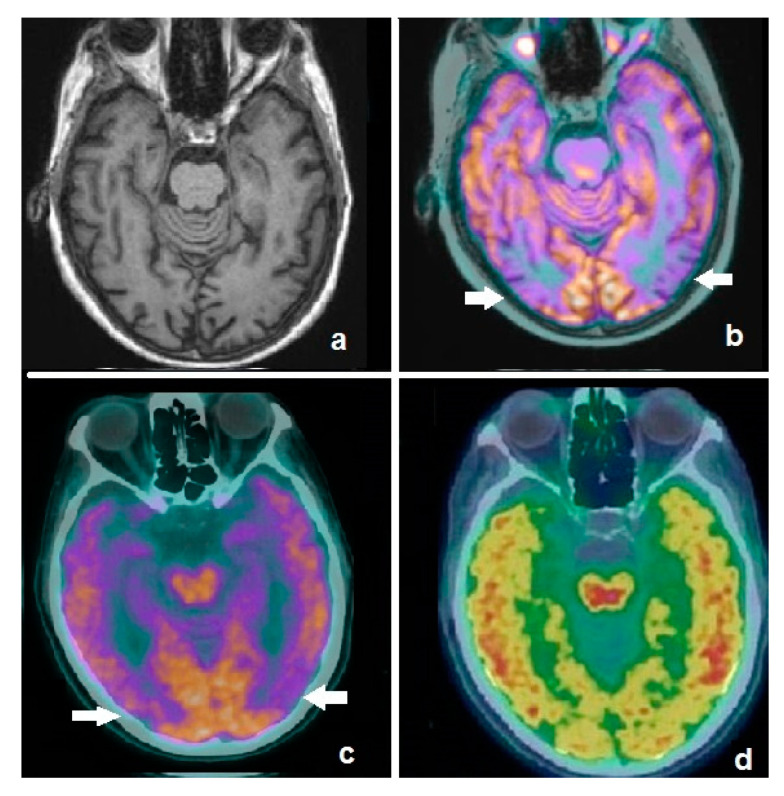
Axial image of an MRI scan in a patient affected by mild cognitive impairment (a), showing no significant abnormalities. Fused corresponding ^18^F-FDG PET/MRI demonstrates hypometabolism in temporo-occipital lobes (b, white arrows). Patient was submitted to dual-phase amyloid PET with ^18^F-flutemetamol: the early phase demonstrates an AD-typical pattern (c, white arrows), substantially overlapping with the ^18^F-FDG findings, while the late phase shows pathological amyloid burden in the cortex (d). Dual-phase amyloid PET resulted to be useful for the simultaneous detection of neuronal dysfunction and amyloid burden.
